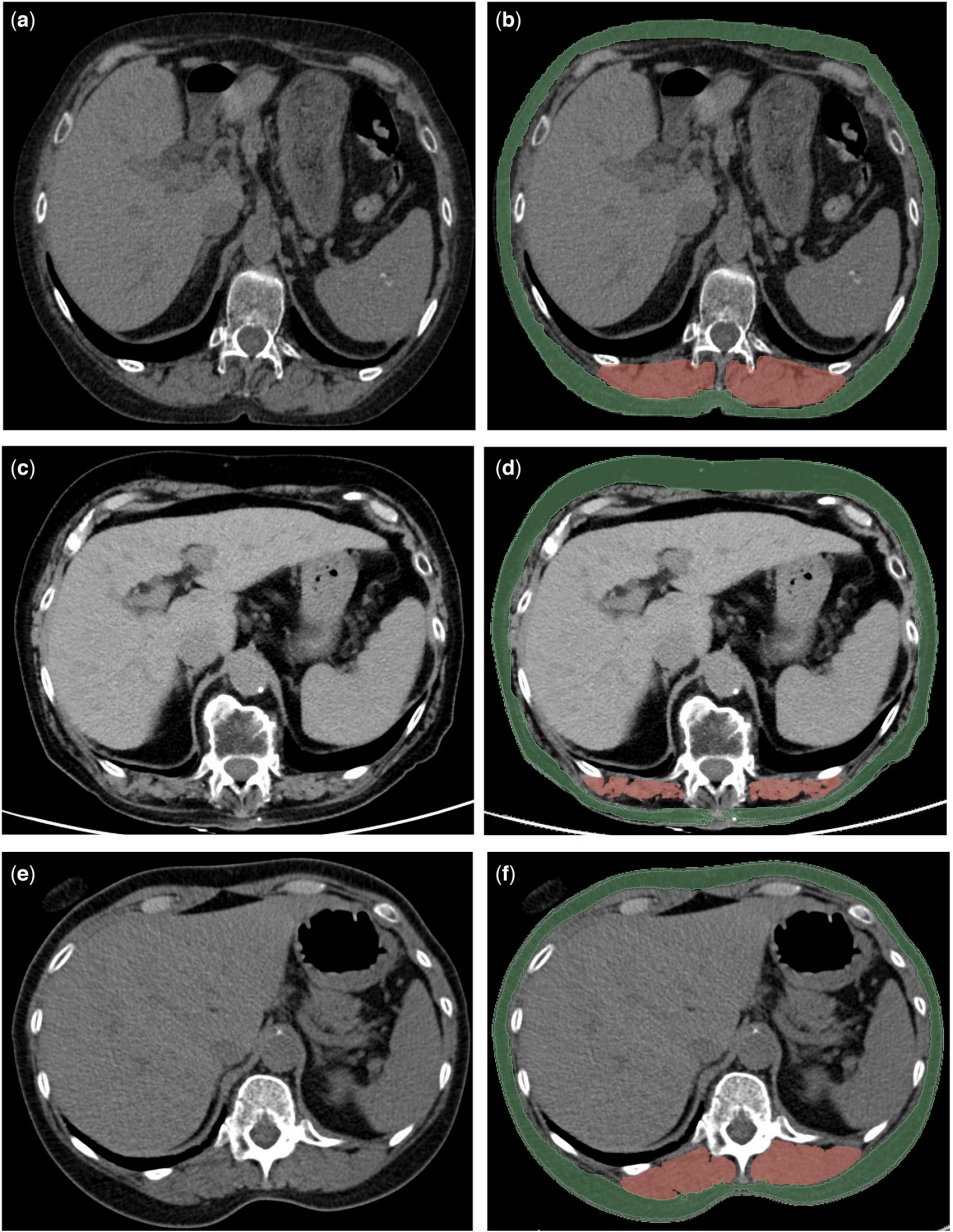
Axial mediastinal window of the high-resolution CT of a 75-year-old female patient with SSc at the level of the 12th thoracic vertebra, used for the body composition evaluation (a). In b, representation of the applied segmentation of the paravertebral muscle (red) and of the subcutaneous fat (green). Axial mediastinal window of the high-resolution CT of a 79-year-old female with limited SSc (lcSSc) at the level of the 12th thoracic vertebra (c) with muscle and subcutaneous segmentation (respectively red and green areas in d) nicely shows the reduced muscle mass and the myosteatosis. Equivalent mediastinal window with the corresponding segmentation of a 54-year-old female with diffuse SSc (dcSSc) (e and f), showing a larger muscle area with preserved muscle density. Moreover, the patient with lcSSc had more hypodense fat tissue (−101 Hu) than the patient with dcSSc (−92 Hu)
